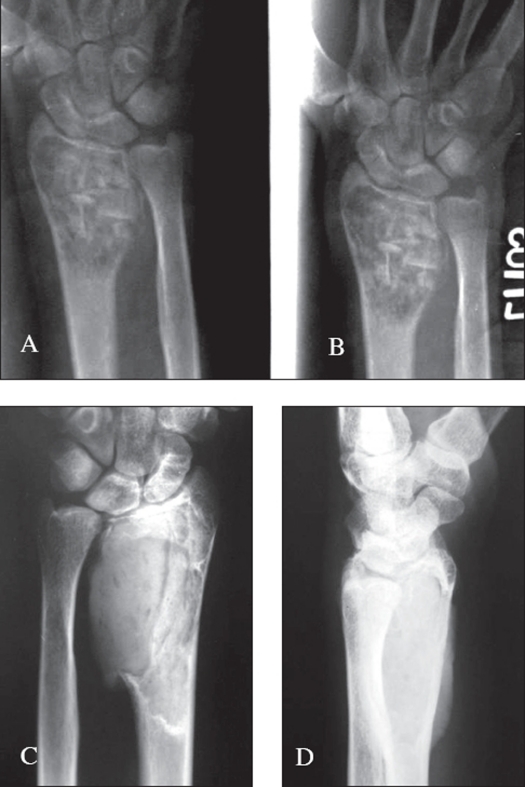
A) Case 1: Grade 2 lesion - Distal radius G.C.T, Treated elsewhere with curettage and bone grafting. B) Had a recurrence, Repeat Curettage and reconstruction done with bone cement. C) Follow up A.P. x-ray at a four year. D) Lateral x-ray of the same patient at four year follow up showing no recurrence of lesion and good subchondral bone
